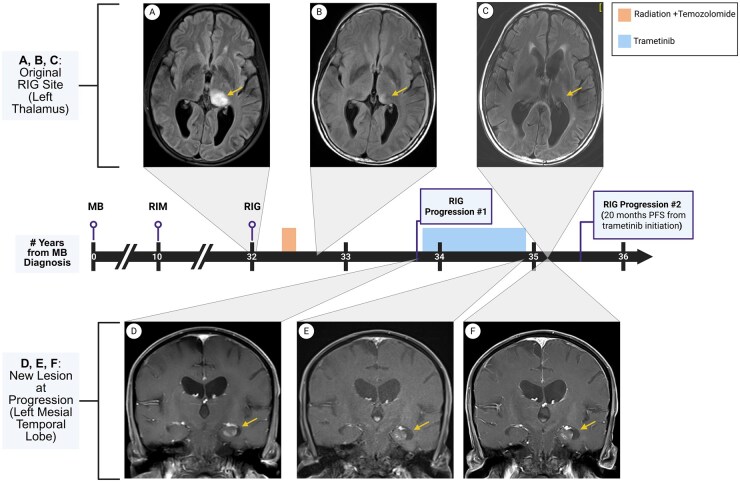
Timeline of diagnosis, treatment, and radiographic response to trametinib. (A) T1-weighted post-gadolinium MRI of the original lesion in the left thalamus at initial presentation, (B) 3 months post-chemoradiation showing treatment response, and (C) 15 months after the initiation of trametinib showing stability. (D) A new lesion in the left mesial temporal lobe was identified 15 months post-chemoradiation, showing disease progression (Progression #1). Treatment with trametinib was initiated, and MRI (E) after 12 months of treatment and (F) 3 months after trametinib discontinuation confirmed stable disease, prior to a second progression that occurred 20 months after trametinib initiation (Progression #2). Abbreviations: MB, medulloblastoma; RIM, radiation-induced meningioma; RIG, radiation-induced glioma; PFS, progression-free survival; MRI, magnetic resonance imaging. Created in BioRender. Schreck, K. (2026) https://BioRender.com/57ob9ut.
